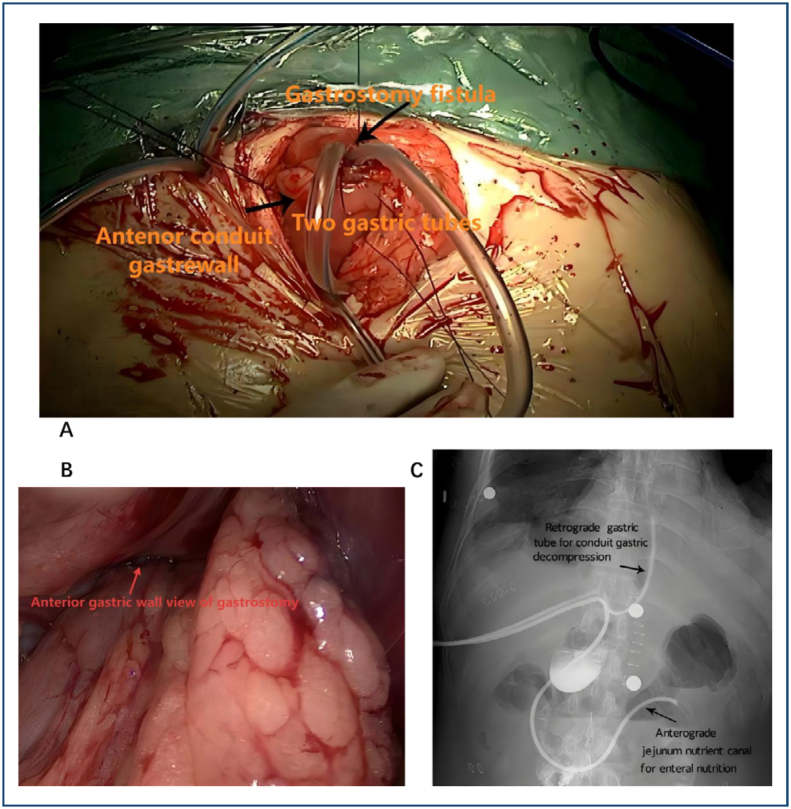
Gross anterior view (A), intraperitoneal view (B), and plain abdominal radiograph (C) after tube placement.
